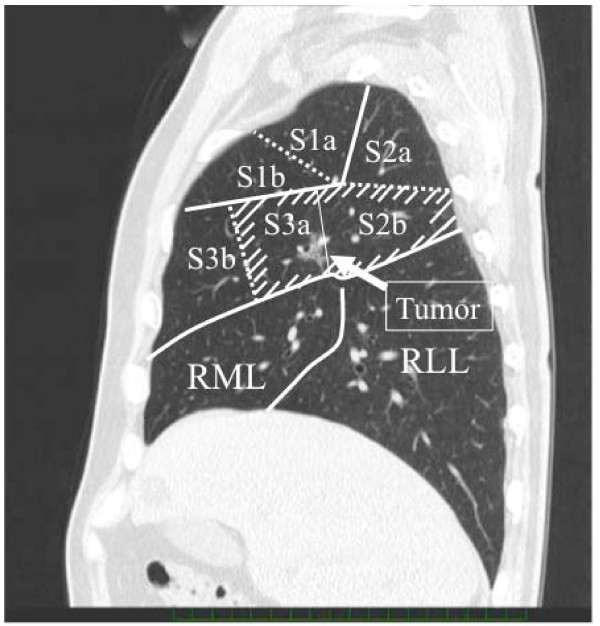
Sagittal image of CT. The tumor located between the right subsegment 2b and 3a.
